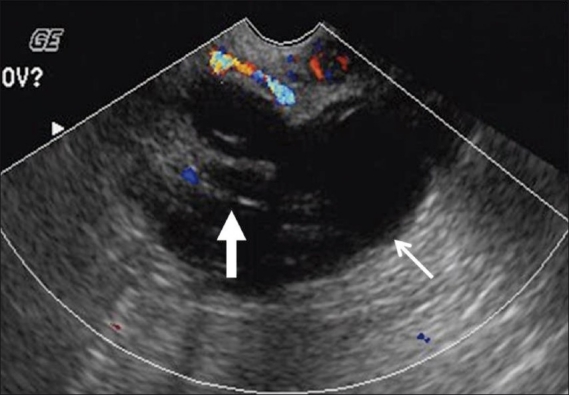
Transvaginal pelvic USG with color Doppler shows a complex cystic mass (thin arrow) with septae and solid components (thick arrow) and no definite intralesional vascularity
